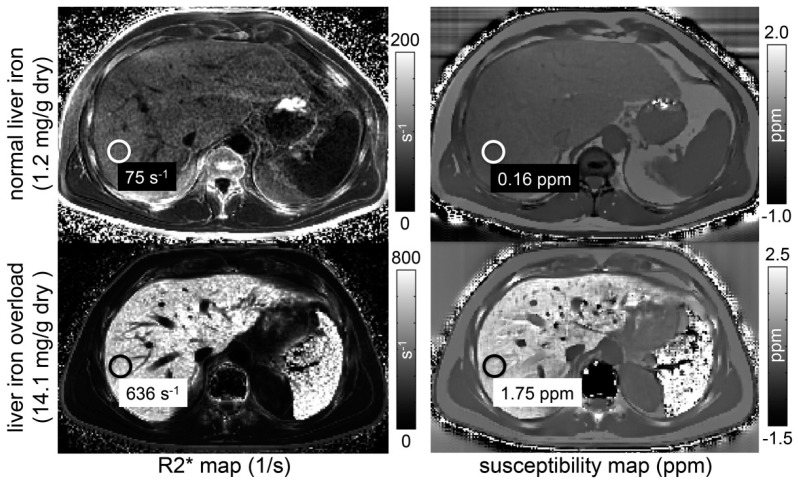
Both MRI−based R2* mapping and quantitative susceptibility mapping are sensitive to the presence of iron in the liver. The figure shows R2* maps (left) and susceptibility maps (right) estimated from a subject with normal liver iron (top) and with liver iron overload (bottom). Super−conducting quantum interference device–biomagnetic liver susceptometry estimates of liver iron concentration are reported. The susceptibility measurements reflect the change in liver susceptibility due to the presence of iron. For the subject with liver iron overload, notice the increase in R2* and susceptibility in the spleen, suggesting the presence of iron overload in that organ as well. Re−produced with permission from Sharma SD et al. [69].
